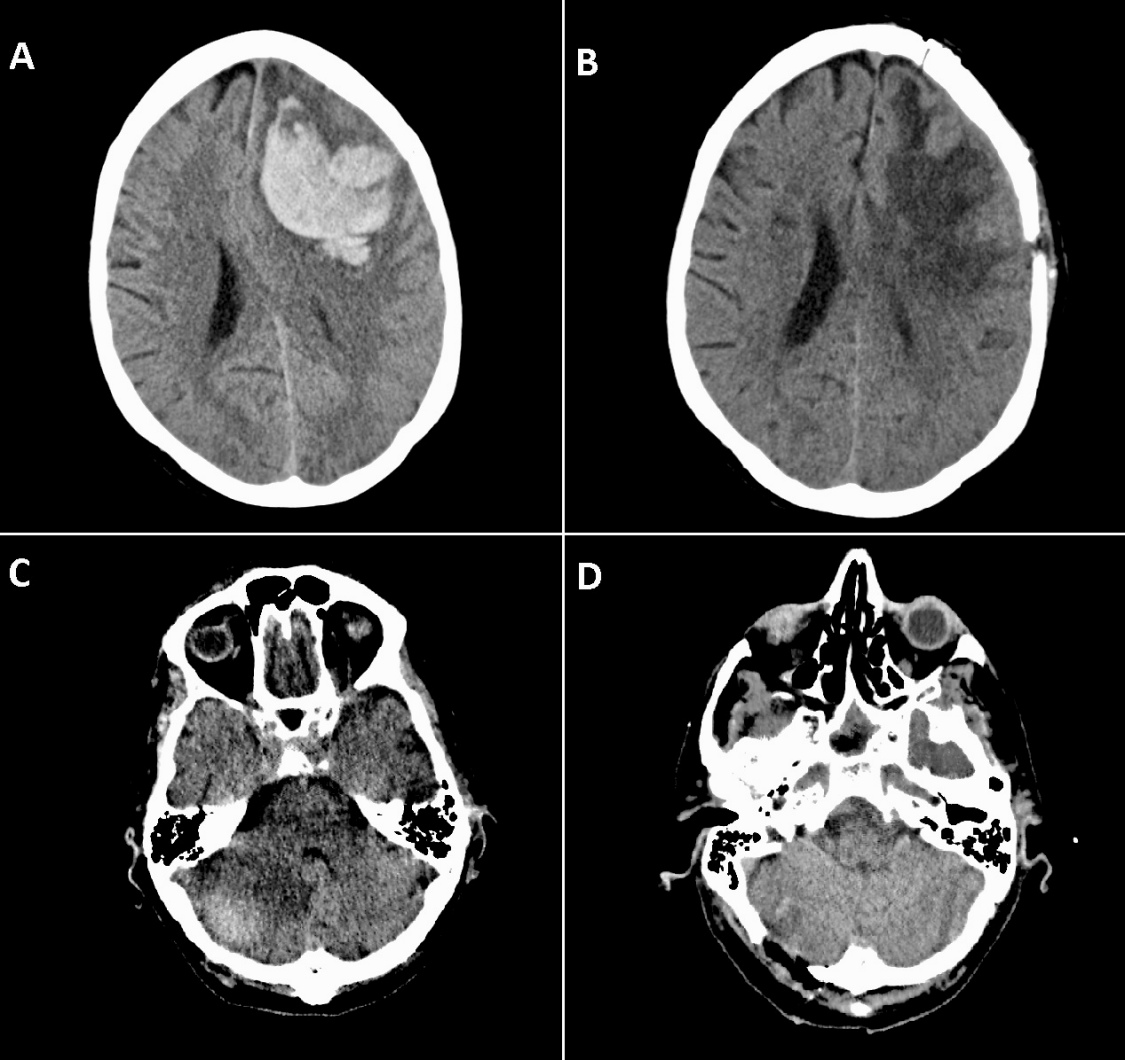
Computed tomography (CT) scans of the patient’s brain (A) Left frontal intracerebral hemorrhage (ICH) preoperatively; (B) Left frontal ICH postoperatively; (C) Right cerebellar ICH preoperatively; (D) Right cerebellar ICH postoperatively.
